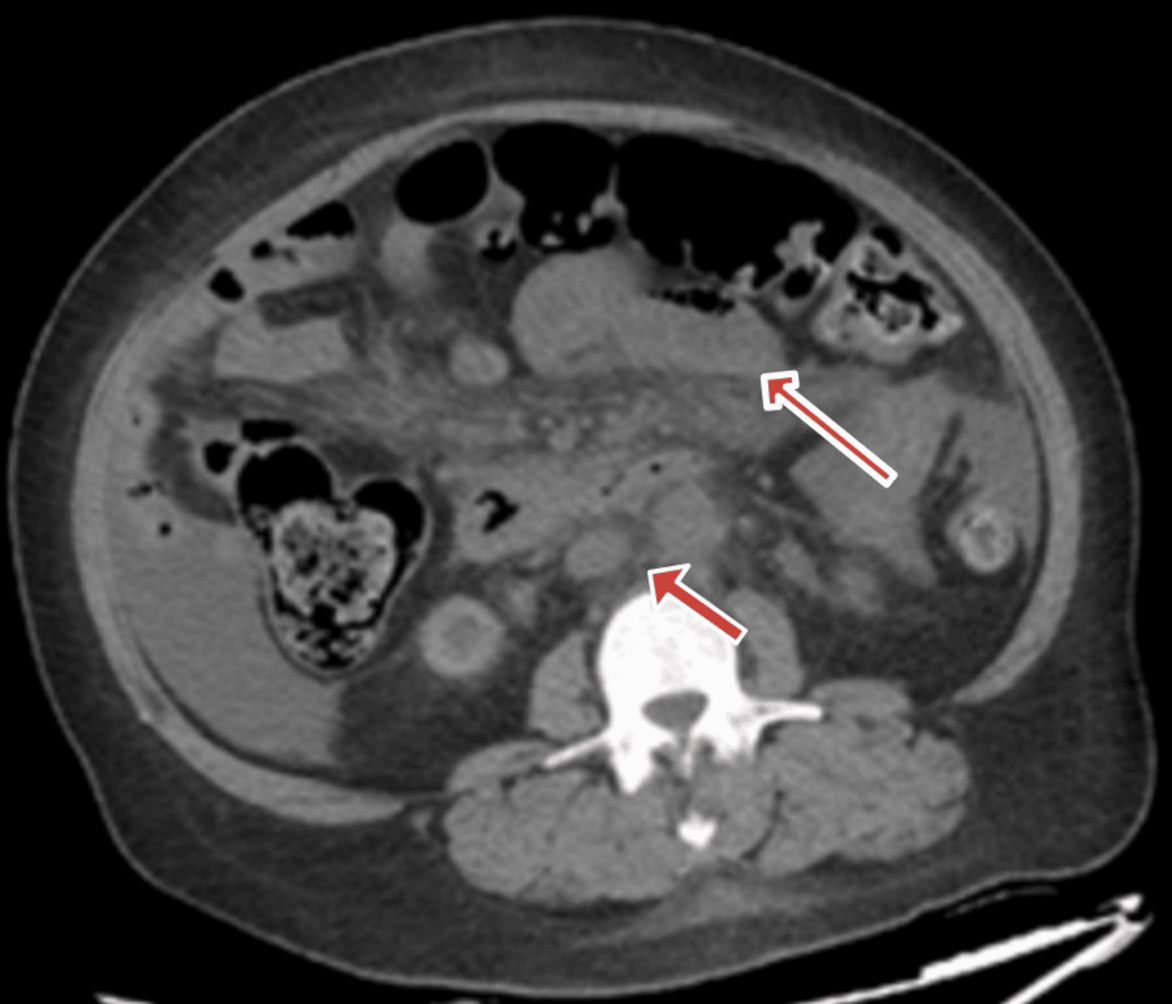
CT scan showing mesenteric lymphadenopathy and close-loop obstruction
